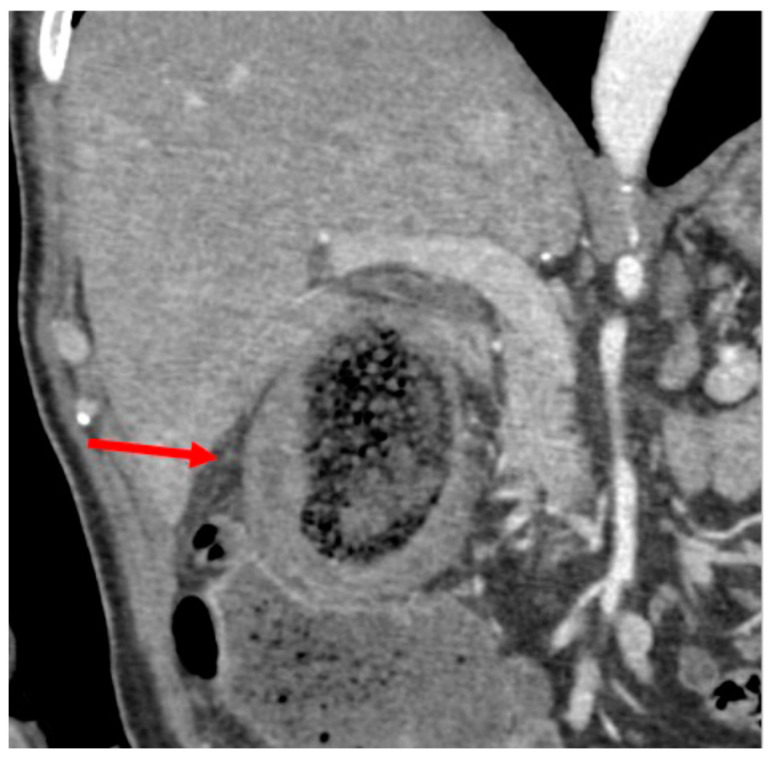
CT scan: thickened gallbladder walls with loss of normal wall stratification (arrow).
